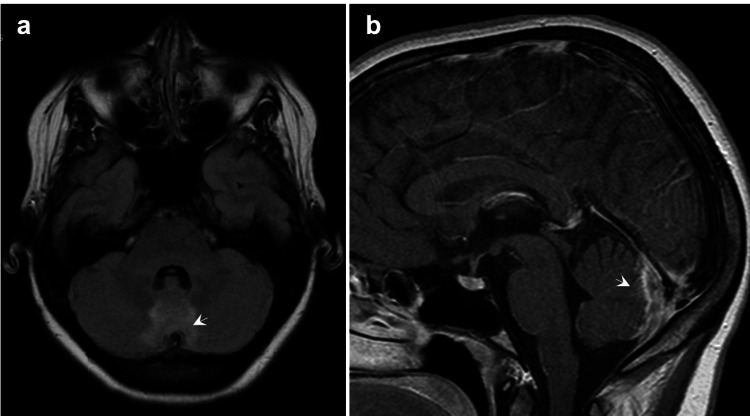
Two weeks later, (a) T2WI shows an increase in hyperintensity in two-thirds of the superior vermis and adjacent areas, indicating an increase in vasogenic edema (arrow). (b) Contrast-enhanced T1WI shows an increase in irregular pachymeningeal enhancement adjacent to the cerebellar vermis, without restricted diffusion (arrow). T2WI: T2-weighted imaging; T1WI: T1-weighted imaging.
